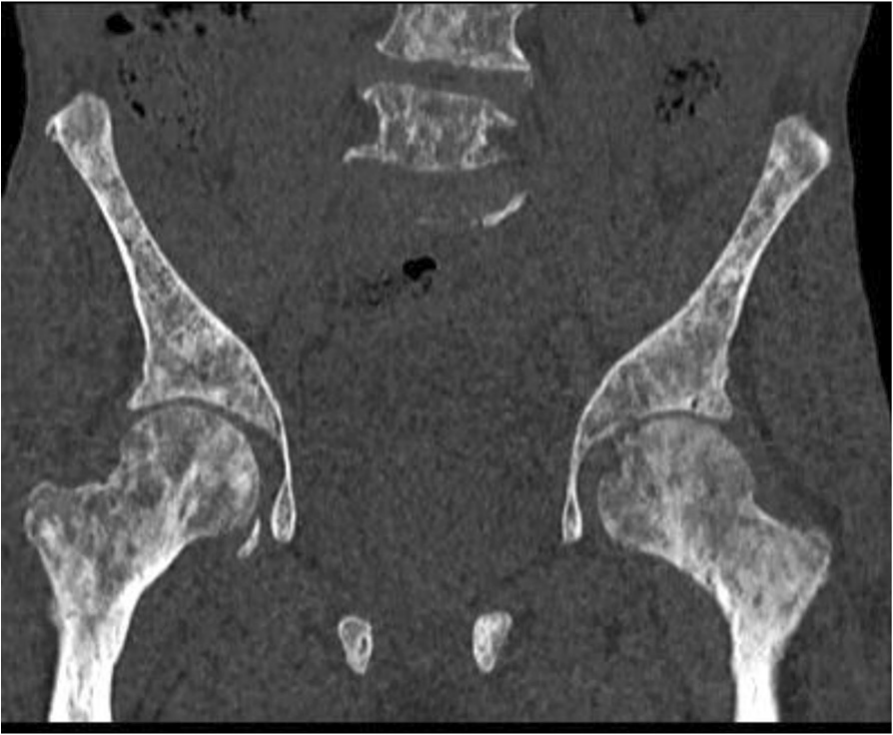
Computed tomography scan showing lytic lesions involving the femur and pelvic bones.
